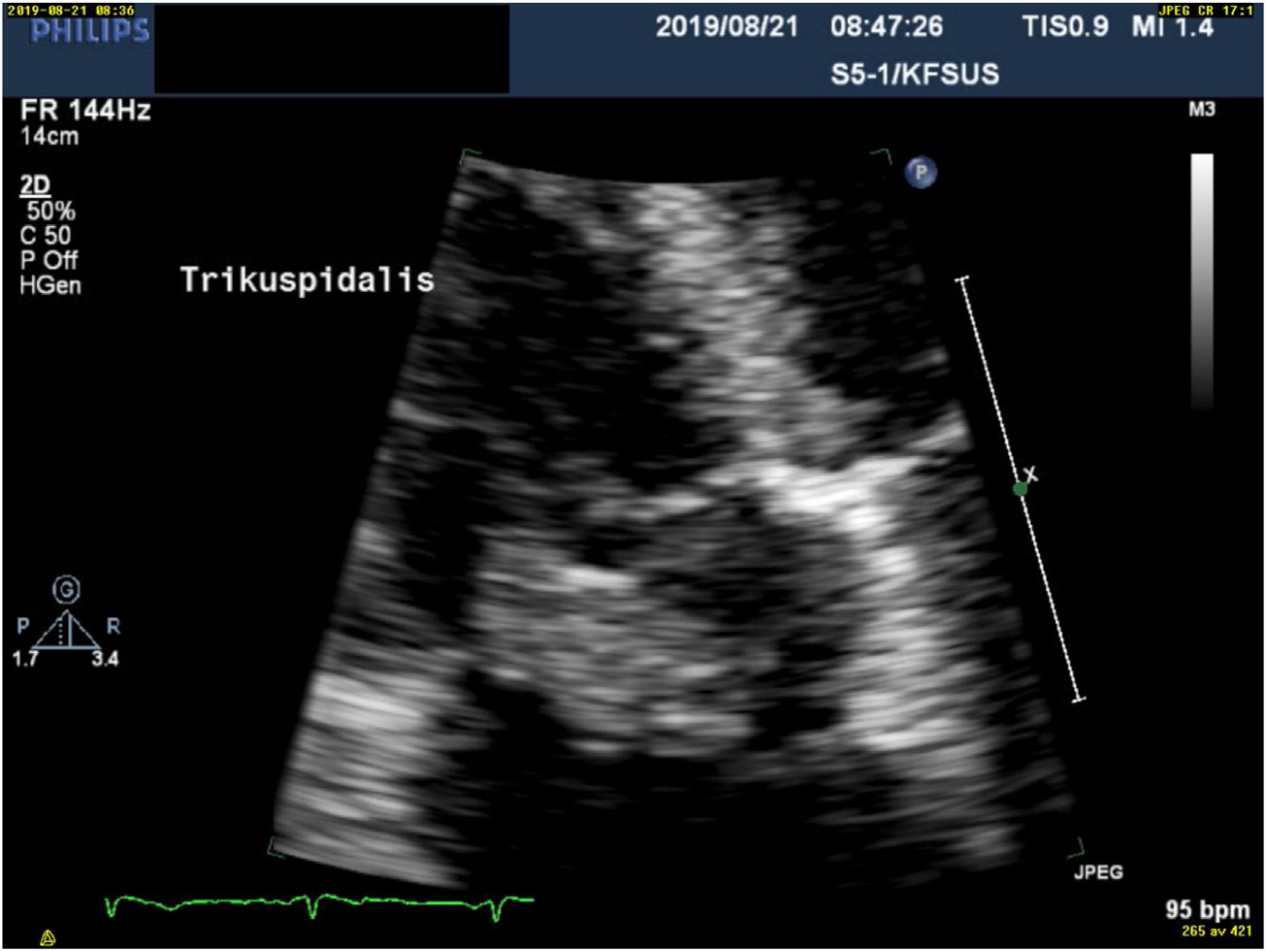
Transhoracic echocardiography demonstrating enlarged tricuspid vegetation.
